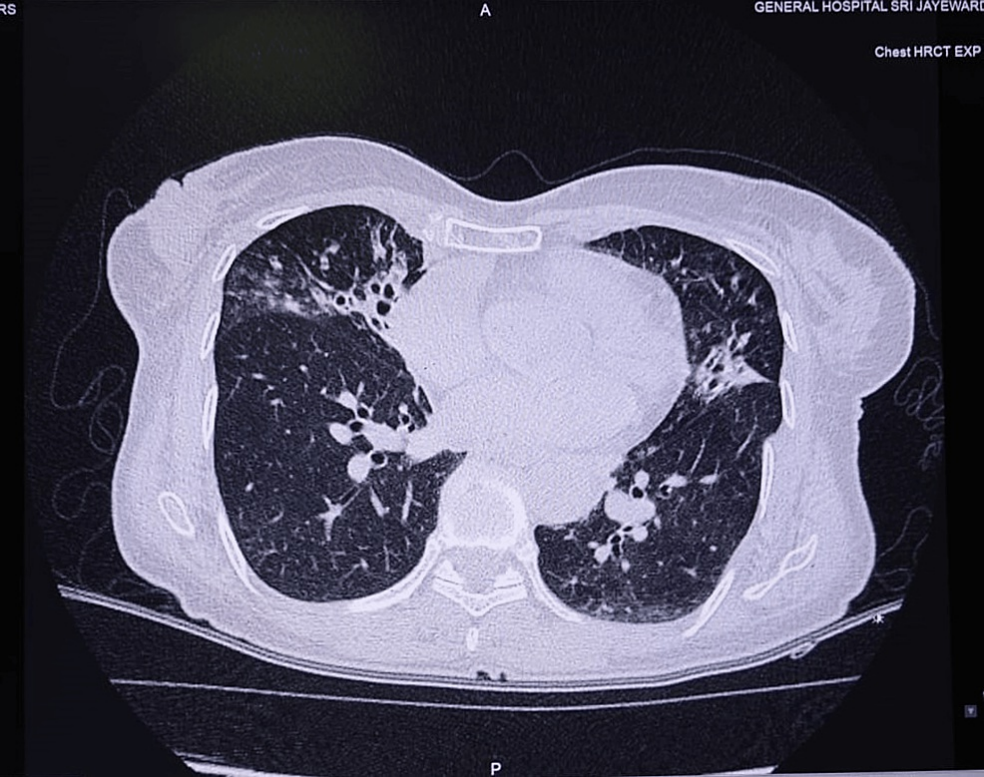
HRCT of the 64-year-old female with intermittent fever and dry cough for one month with recent-onset hemoptysis (axial view) HRCT: high-resolution CT
